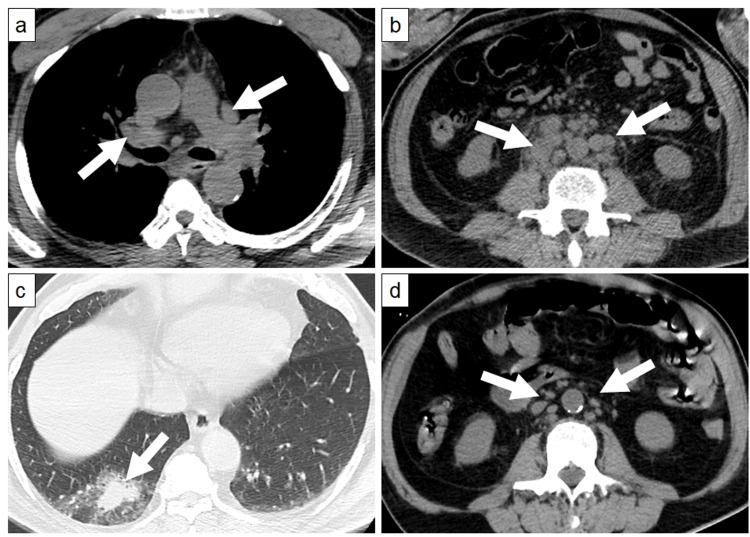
Non-contrasted CT images at hospital admission and on the day of death (a) A non-contrasted CT at admission shows enlargement of hilar and mediastinal lymph nodes (arrows), (b) enlargement of para-aortic lymph nodes (arrows), and (c) consolidation in the right lower lobe (arrow). (d) On the day of death, a non-contrasted CT reveals a reduction in the size of the para-aortic lymph nodes (arrows) compared to the CT at admission (b).
